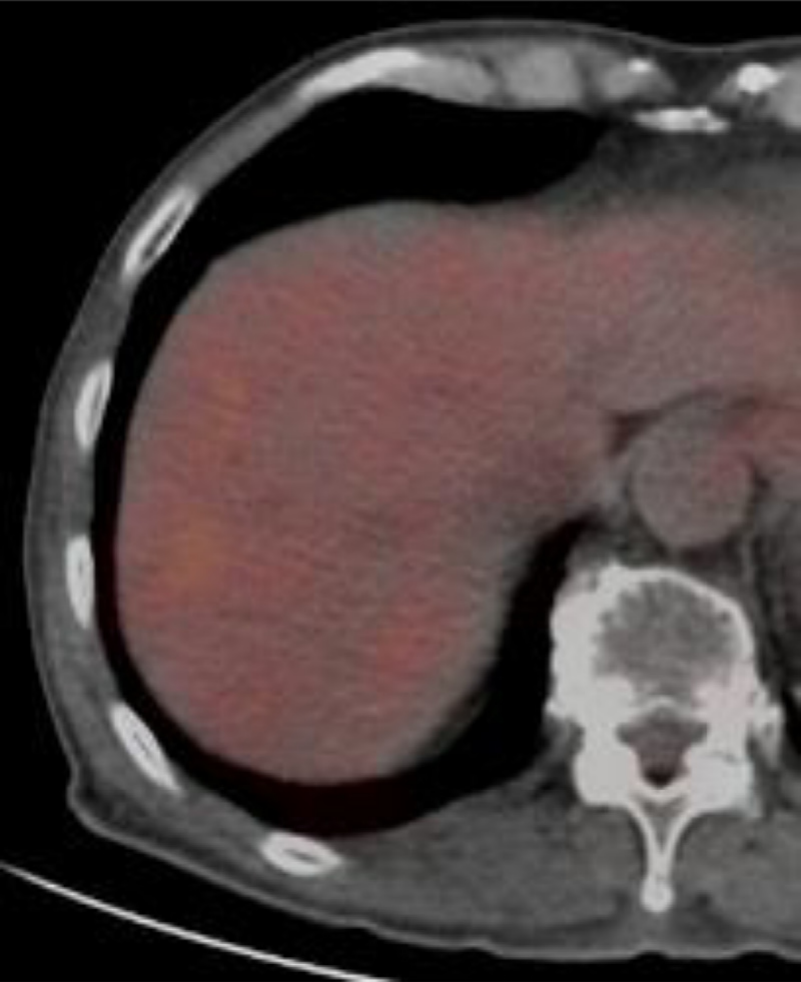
Positron emission tomography (PET) /CT PET/CT showed no fluorodeoxyglucose accumulation in the liver.
